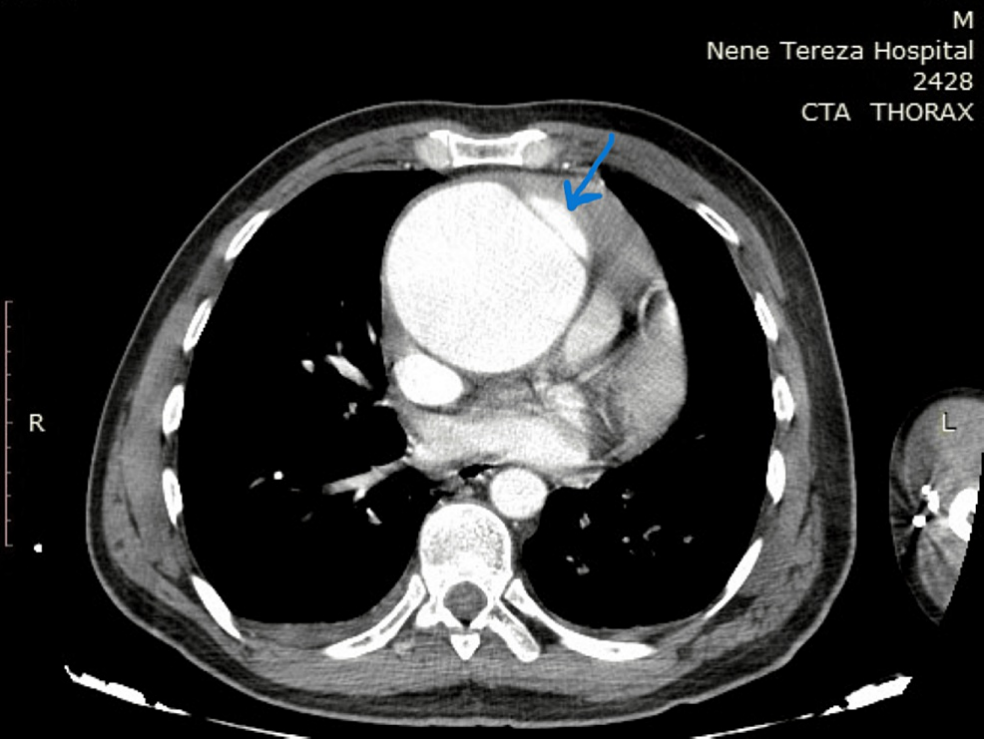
Computed tomography with intravenous contrast The blue arrow indicates the flap dissection.
